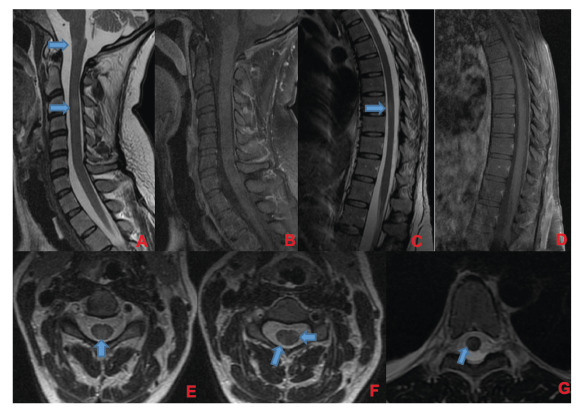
Corresponding spinal cord images of MRI shown in Figure 2. Demyelinating lesions in cervical (A, B, E, and F) and thoracic spinal cord (C, D, and G). In A and B, sagittal plane of cervical spinal cord, showing lesions in T2-weighted image (A) without enhancement in T1 post-gadolinium (B). There are involvement of spinal cord grey matter (E) and white matter (F) as shown in axial T2-weighted images. In C and D, sagittal plane of thoracic spinal cord, showing one lesion in T2-weighted image *(C)* without enhancement in T1 post-gadolinium (D). This lesion was located in spinal cord white matter as shown in axial T2-weighted image in that level (G). Demyelinating lesions are indicated by blue arrows.
